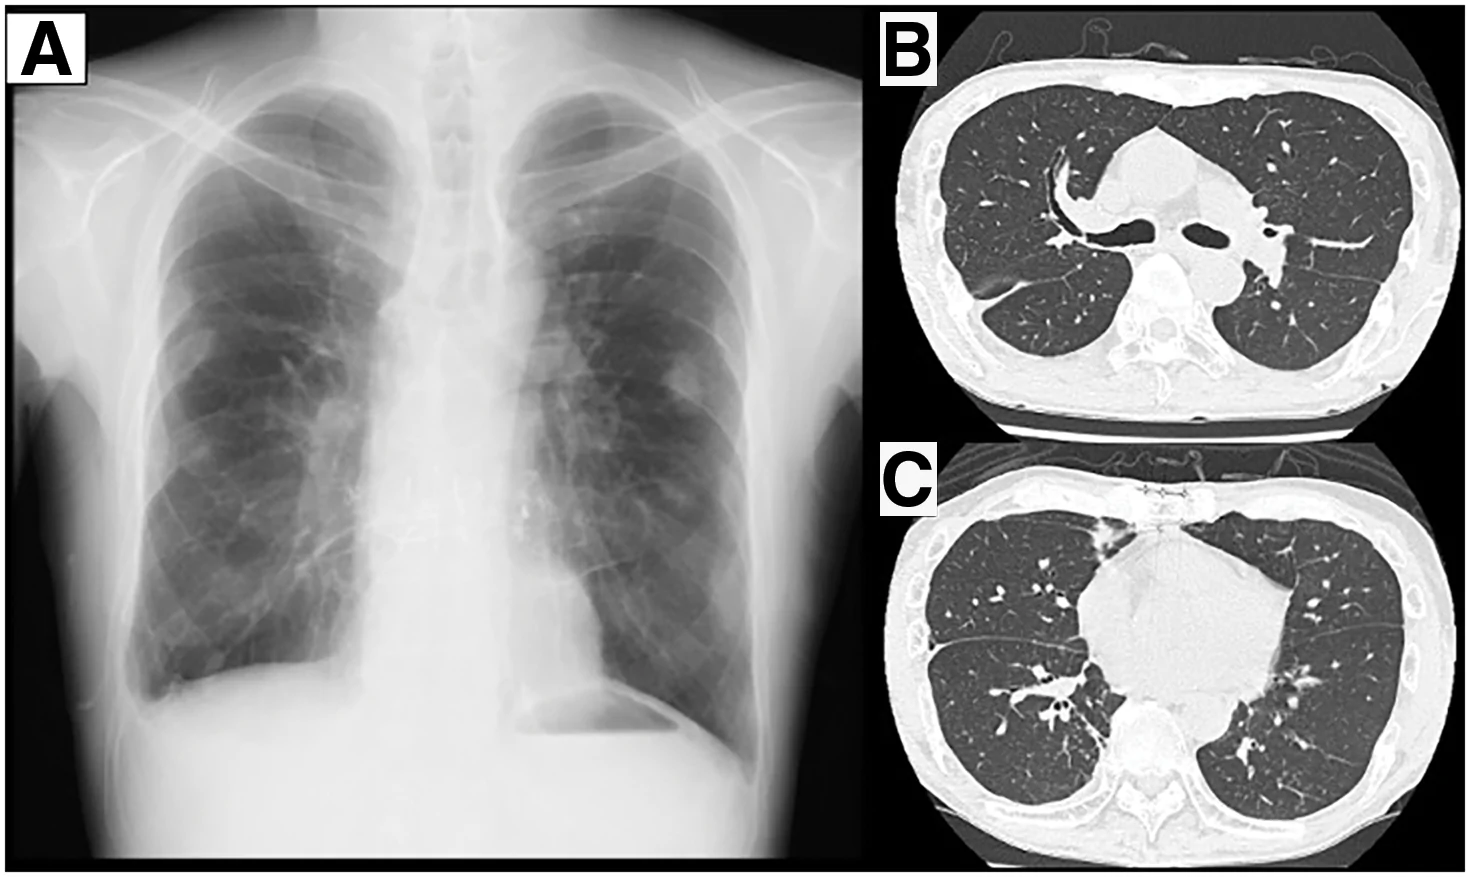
Chest radiograph (A) and CT (B, C) at hospital discharge showing well-expanded bilateral lung grafts without any graft-related complications.
